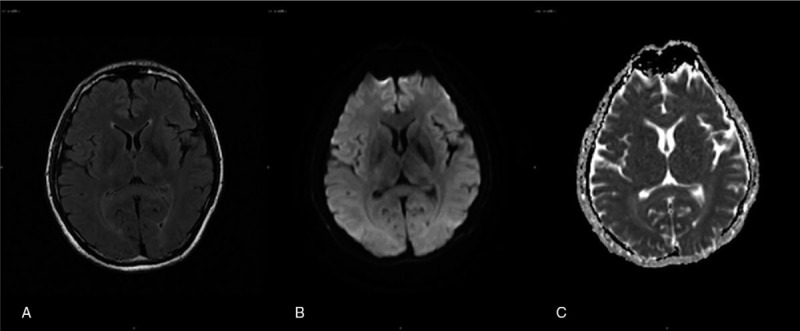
Follow-up MRI after cessation of metronidazole for 1.5 mo. (A) The abnormal findings on T2-weighted FLAIR imaging completely disappeared. (B) The abnormal findings on DWI disappeared. (C) The abnormal findings on ADC disappeared. ). MRI = magnetic resonance imaging; FLAIR = fluid attenuation inversion recovery  ; DWI = diffusion weighted imaging  ; ADC = apparent diffusion coefficient  .
